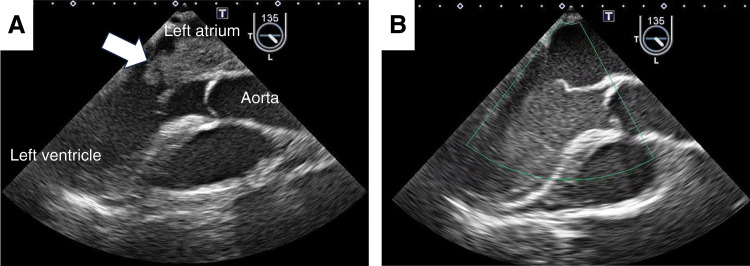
(A) Preoperative TEE shows a mass occupying the entire left atrial cavity (white arrow), with tumor entrapment at the mitral valve. (B) The tumor was no longer detected within the visual field of postoperative TEE. TEE, transesophageal echocardiography
